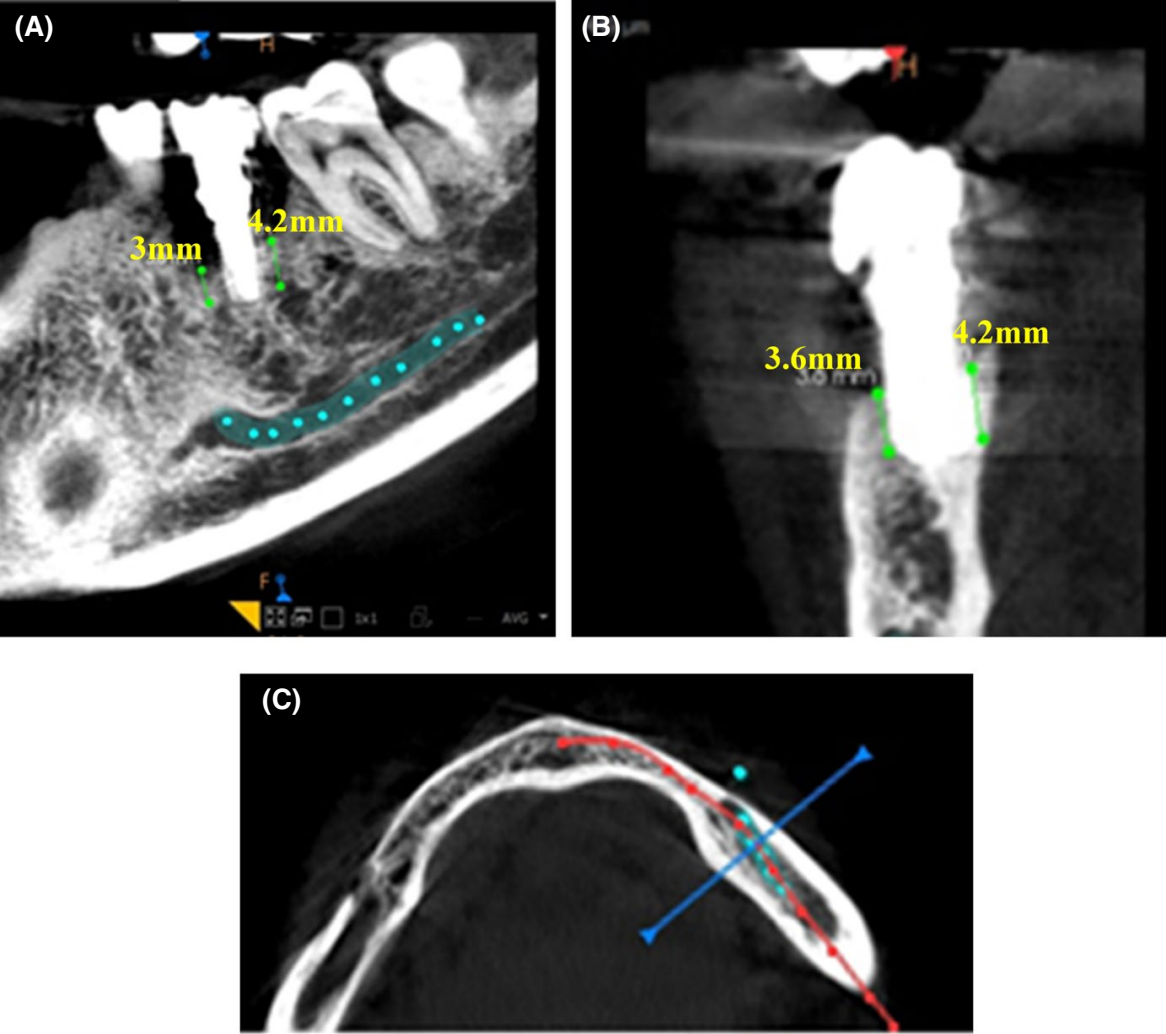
The CBCT imaging of a peri‐implantitis case showing bone resorption in mesiodistal view (A), buccolingual view (B) and axial view (C) around dental implant
